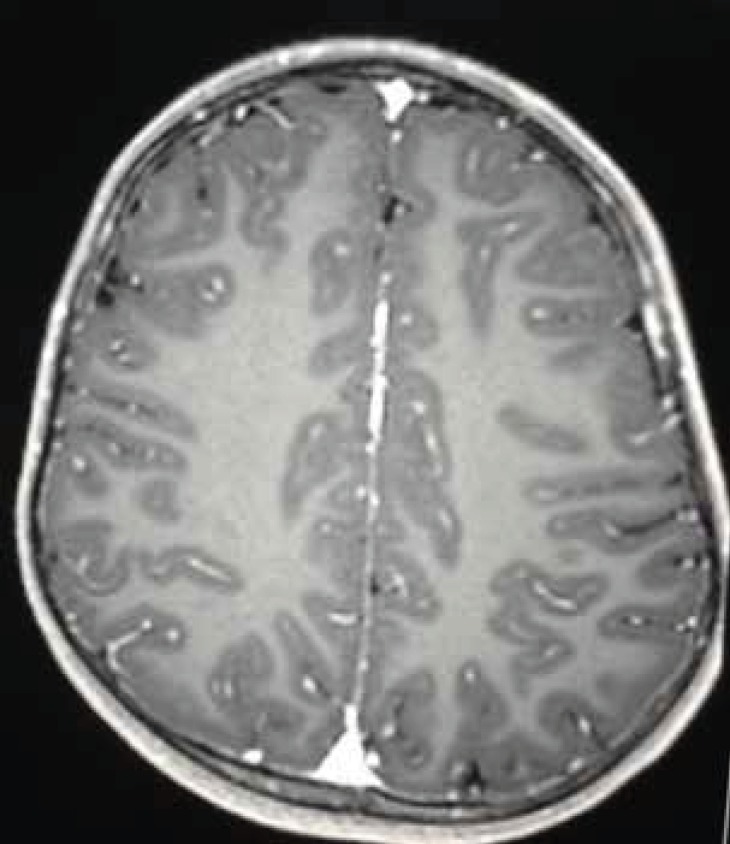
Axial T2 - A repeated MRI after 6months showing complete resolution
